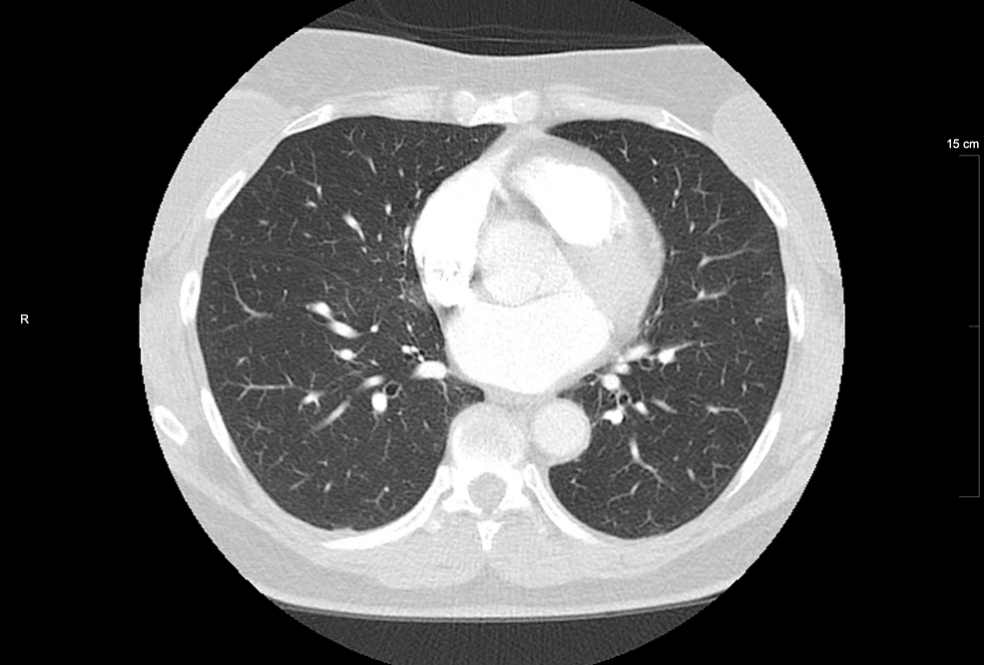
CT angiogram of lung showing atelectasis.
